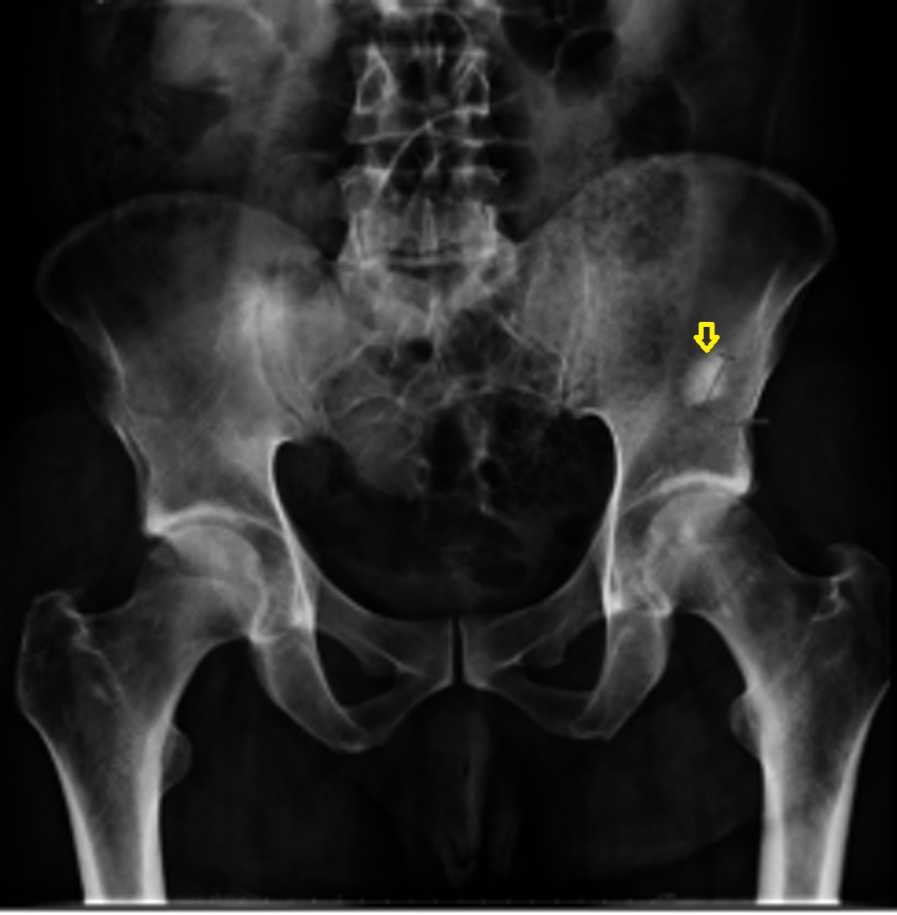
Hip X-ray showing osteoblastic lesion involving the left iliac bone (arrow).
